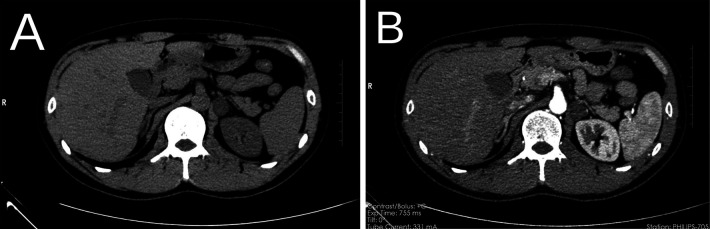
Preoperative CT, left retroperitoneal mass, plain CT (A), contrast-enhanced CT scan (B). CT, computed tomography.
